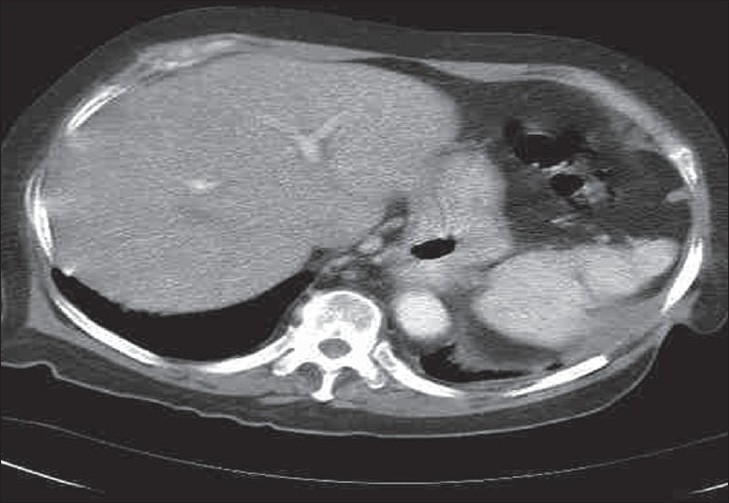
A computed tomograph in the transverse plane, demonstrating a collection of fluid lateral to the spleen
